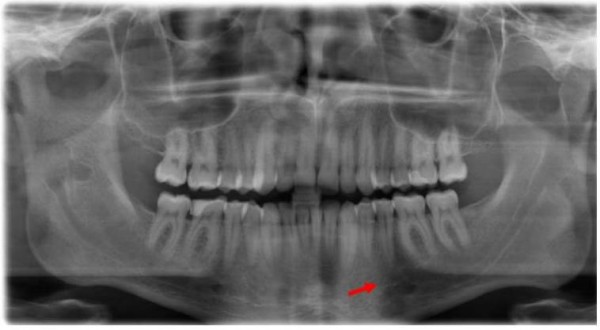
Panoramic radiography (10 month after resection): No recurrence and obvious ossification in the area of the former osteolysis (red arrow).
